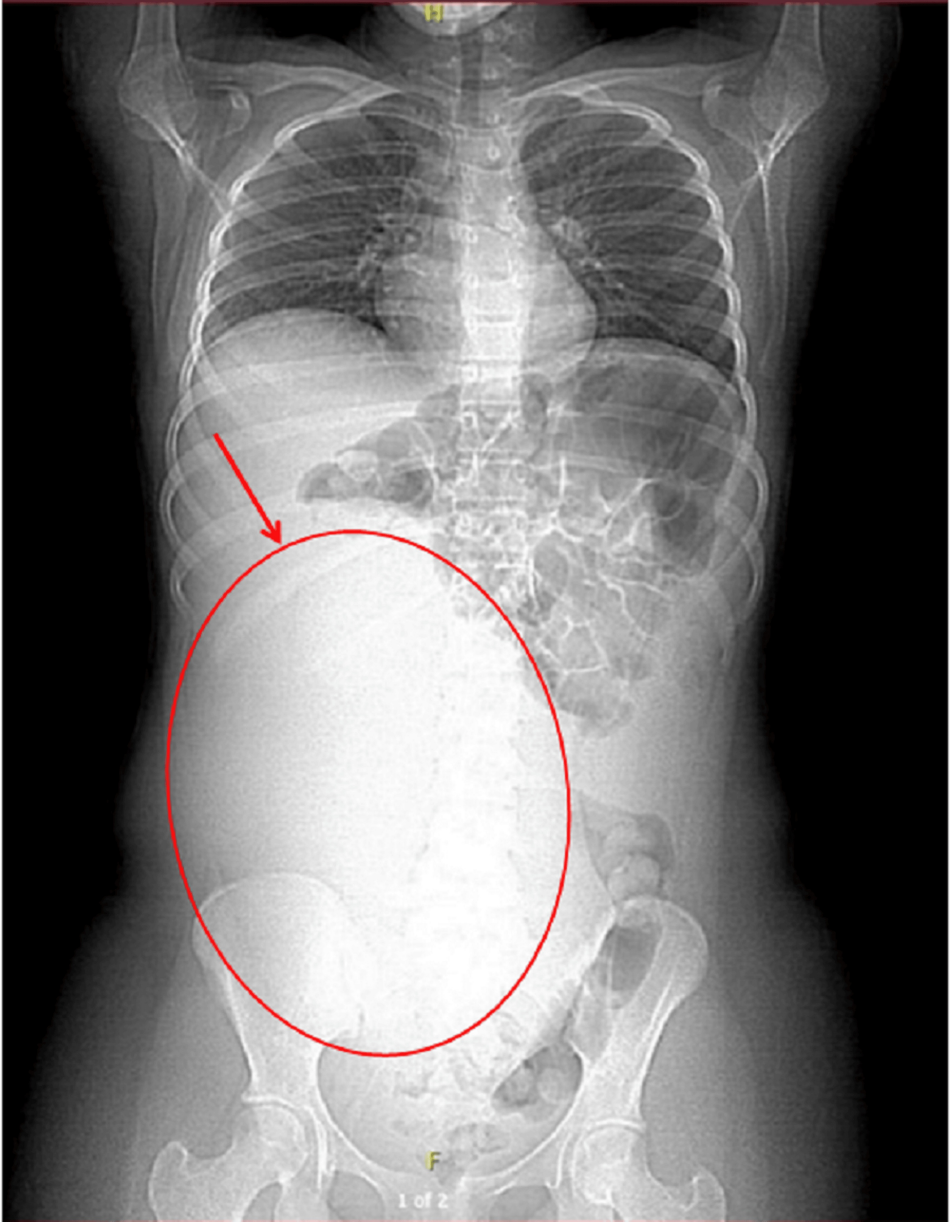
Upright abdominal X-ray showing mass displacement (Case 2)
